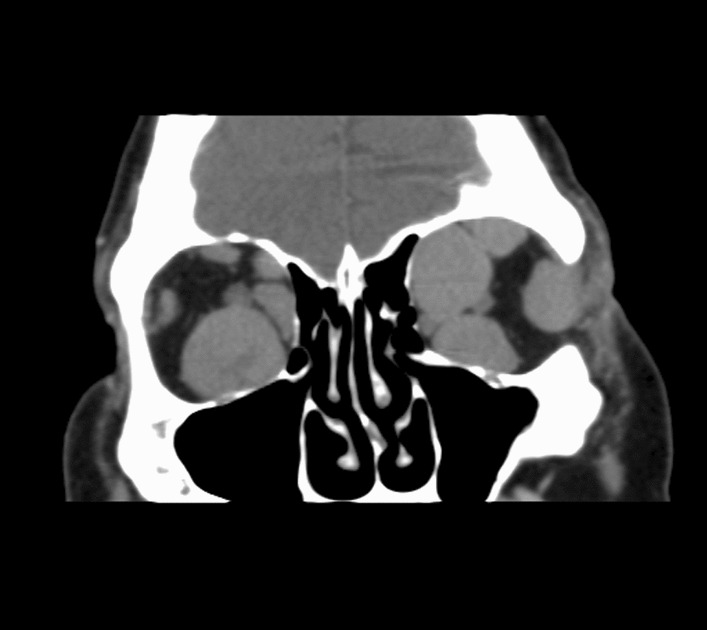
Orbital computed tomography (axial) revealing extraocular muscle infiltration
